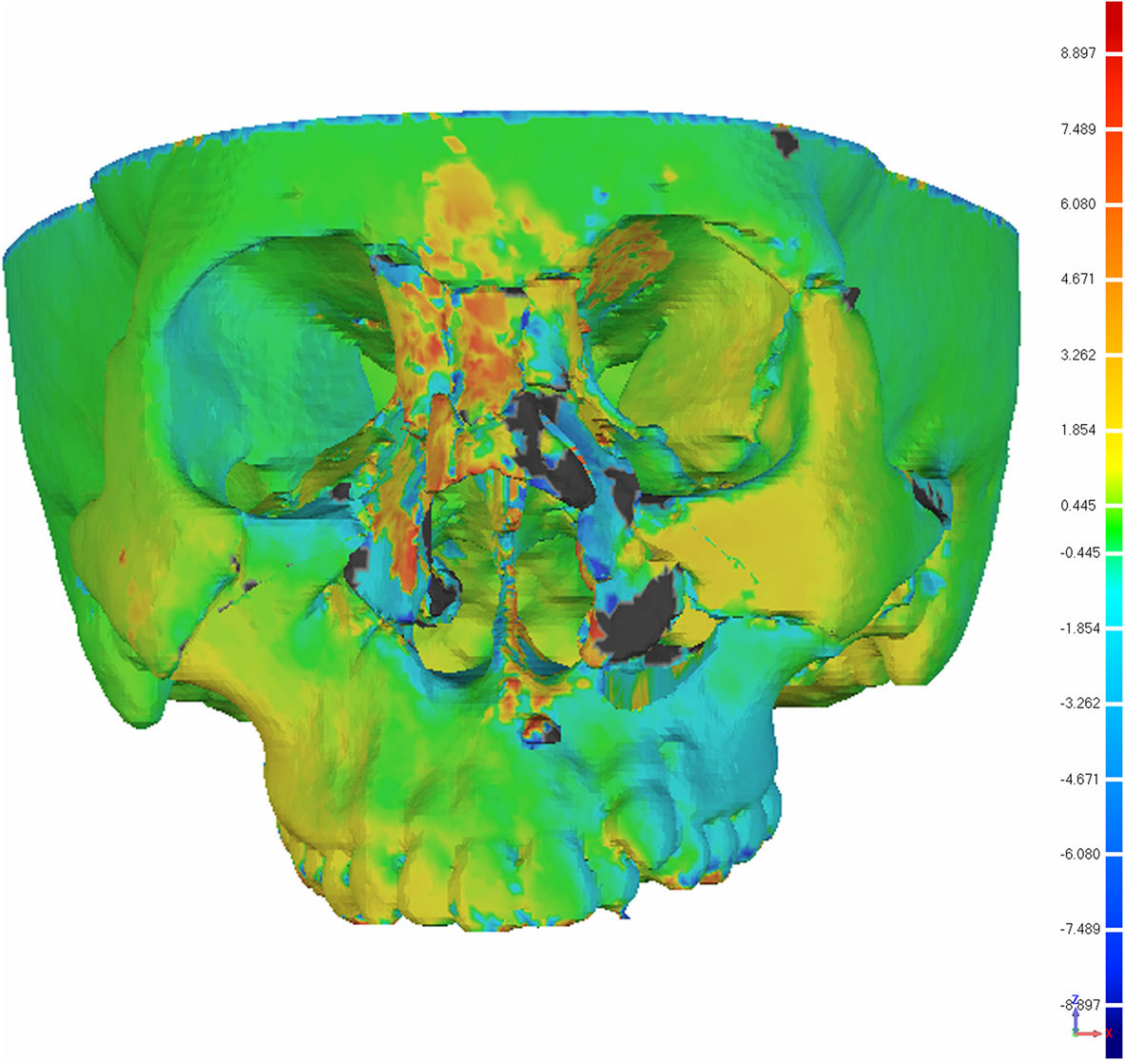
Accuracy of the key points between preoperational surgical plan and 1-week post-operation 3D CT results. The green indicates the most matched area, and the dark blue and red presents the mismatched area
